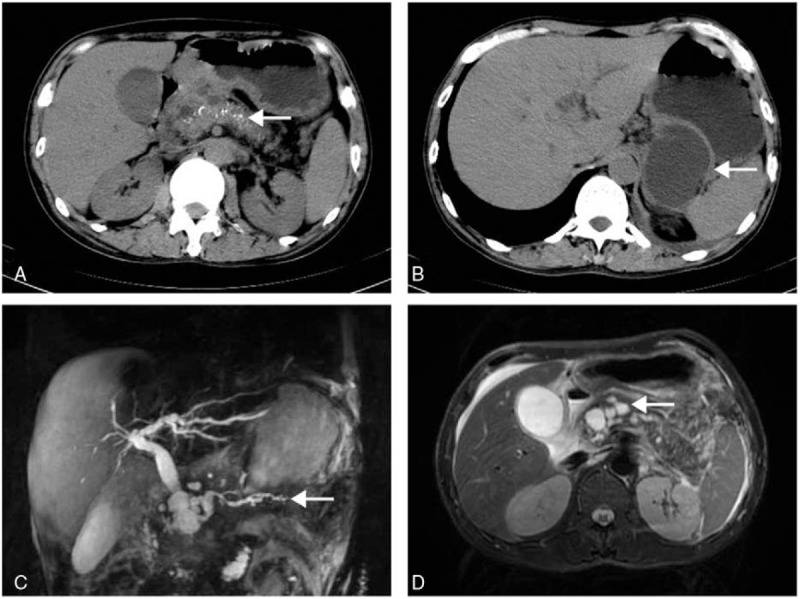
Abdominal CT and MR taken on admission. A, CT scan shows chronic pancreatitis and calcification of the whole pancreas. B, CT scan reveals a 6.5 × 5.7 cm cystic lesion next to the tail of the pancreas. C, MRCP shows irregular dilation of pancreatic duct and dilation of bile duct. D, MR scan reveals multiple small cystic lesions in the head of the pancreas. CT = computed tomography, MR = magnetic resonance, MRCP = magnetic resonance cholangiopancreatography.
